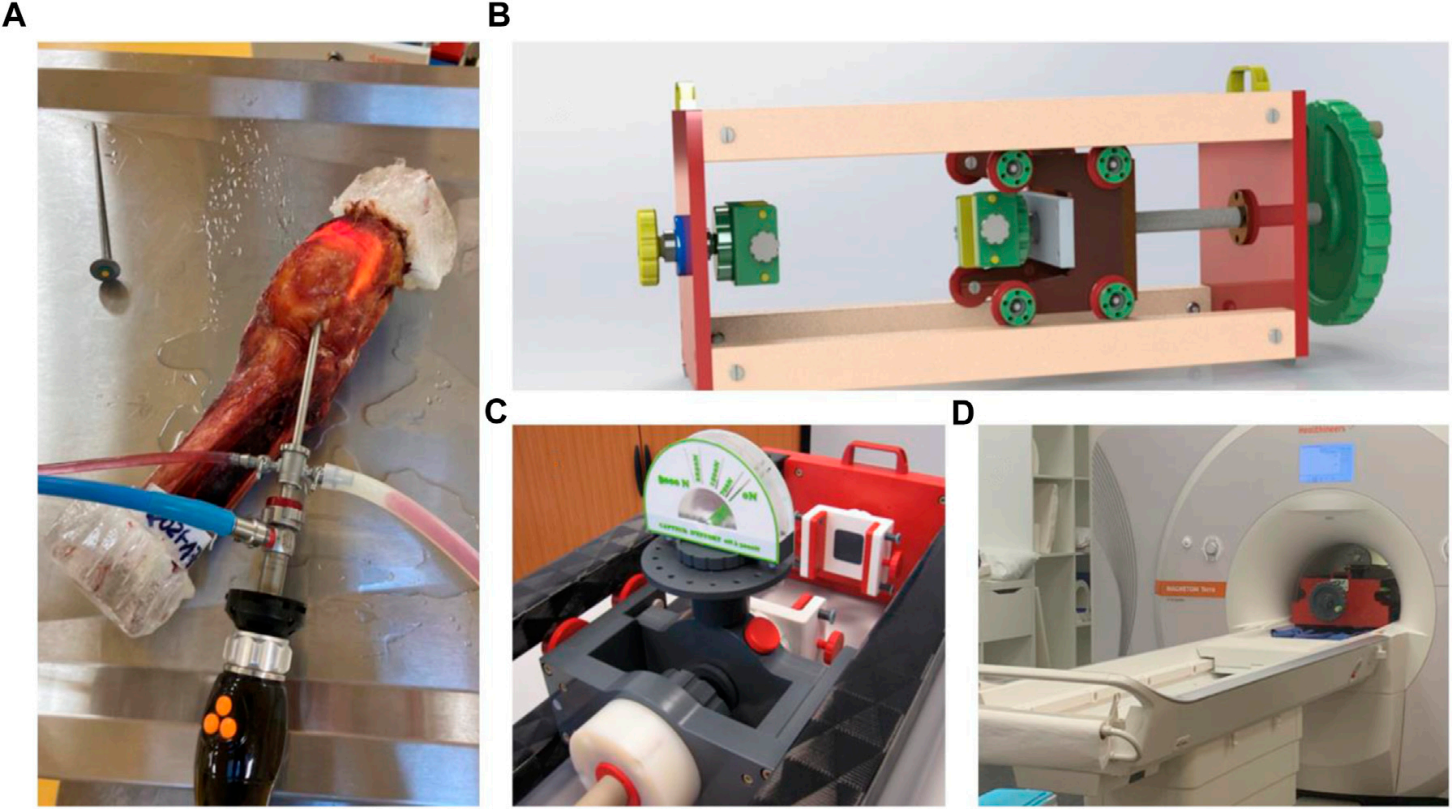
Anatomical view of a knee segment with its proximal and distal fixations of rigid polyurethane resin (A). MRI-compatible loading bench, (B) pressure sensor, (C)
